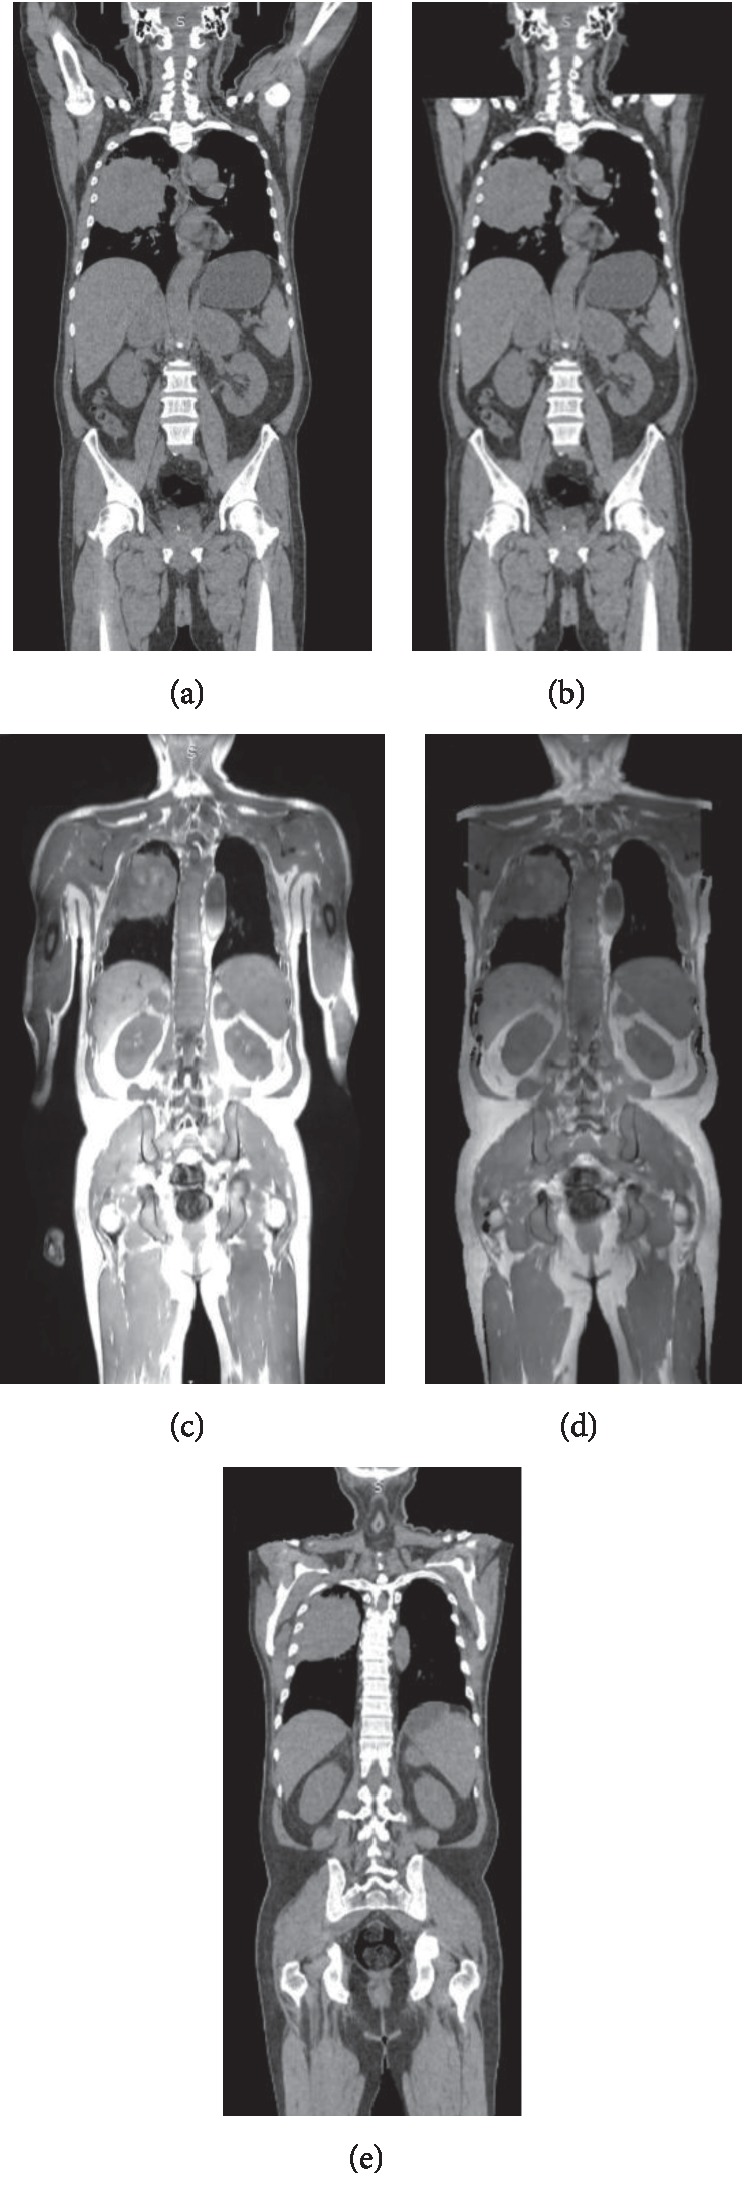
Coregistration process between MR and CT images. (a) Whole-body CT image; (b) CT image without arm; (c) whole-body MR image; (d) MR image without arm; (e) CT image that coregistered to MR image space.
